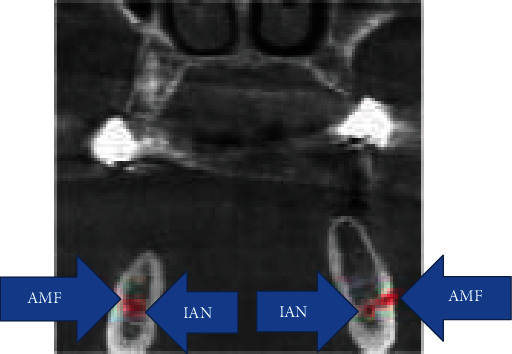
Right and left AMF at coronal CBCT.
